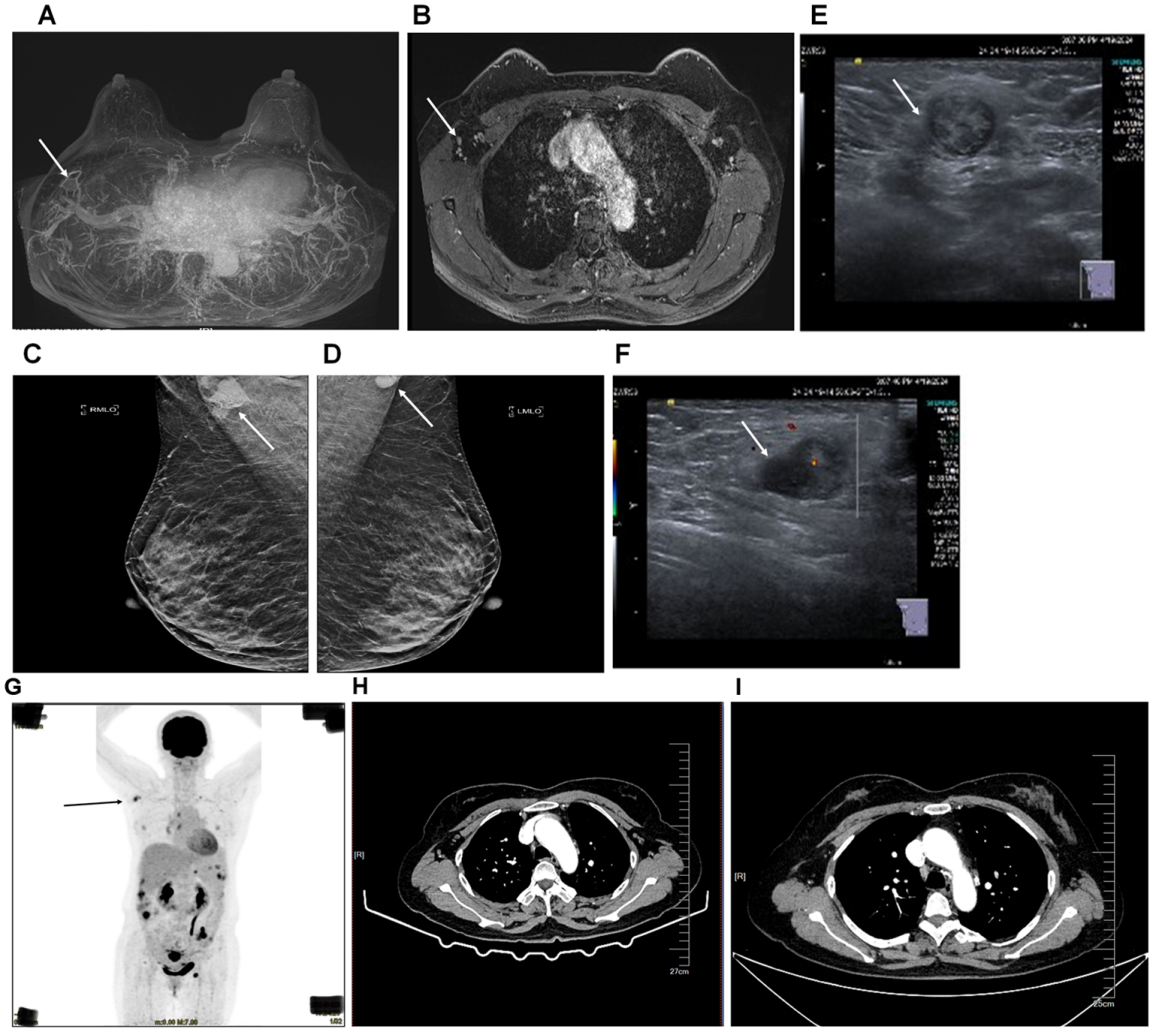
Imaging diagnosis of breast cancer and diagnostic imaging of axillary lymph node and ovarian tumor before and after surgery. (A, B), MRI of the patient’s breast. (C, D), mammography of the patient’s breasts. (E, F), breast ultrasound of the patient’s lymph node. The arrow pointed at the enlarged lymph node in the right axilla. (G) PET-CT scan showing FDG-avid nodules throughout the body (black arrow), with significant uptake in the axillary lymph node and right axilla. (H) Enhanced chest CT scanning of the patient before surgery. The arrow pointed at the lymph nodes in the right axilla. (I) Enhanced chest CT scanning of the patient 4 months after surgery. The arrow pointed at the lymph node in the right axilla.
